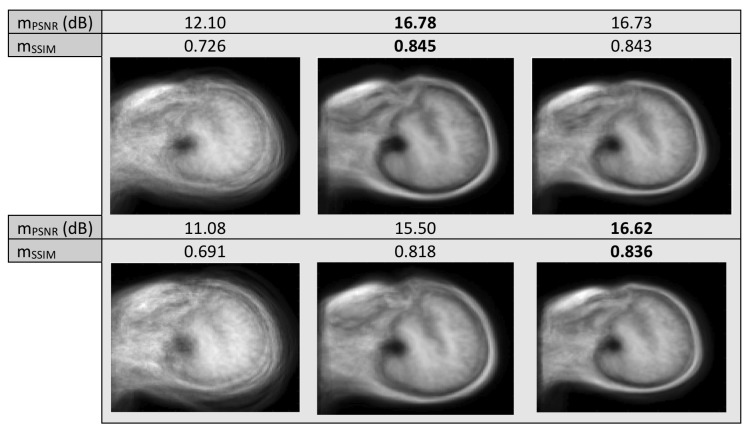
Mean misalignment images of MR images of the same modality with no warp (first row) and with warp of σ=5 (second row). Images before alignment (first column) and after alignment with LKE (second column) and the proposed (third column).
